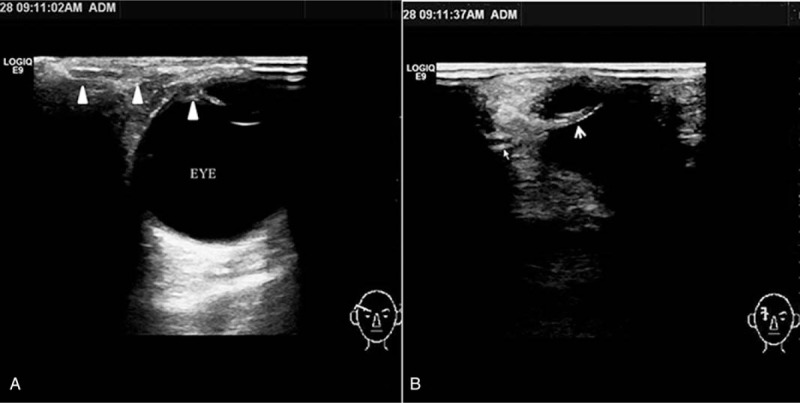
(A) Ultrasound showing the position of the drainage catheter (white triangle) in the horizontal direction. (B) Ultrasound showing the position of the drainage catheter (white arrow) in the vertical direction.
